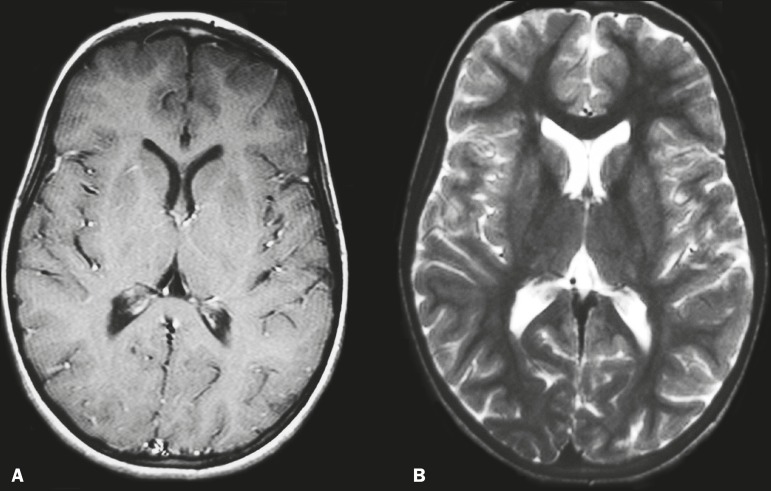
Follow-up MRI of the brain, three months after the first, showing regression
